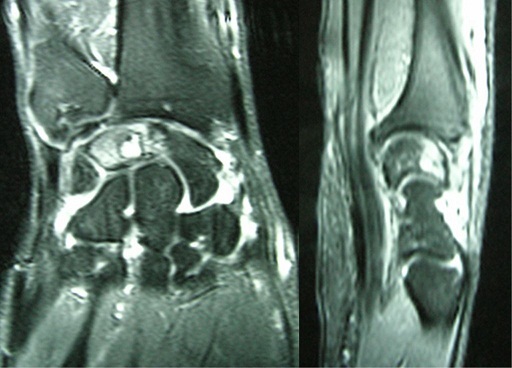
MRI, T2-weighted image and MRI arthrogram
